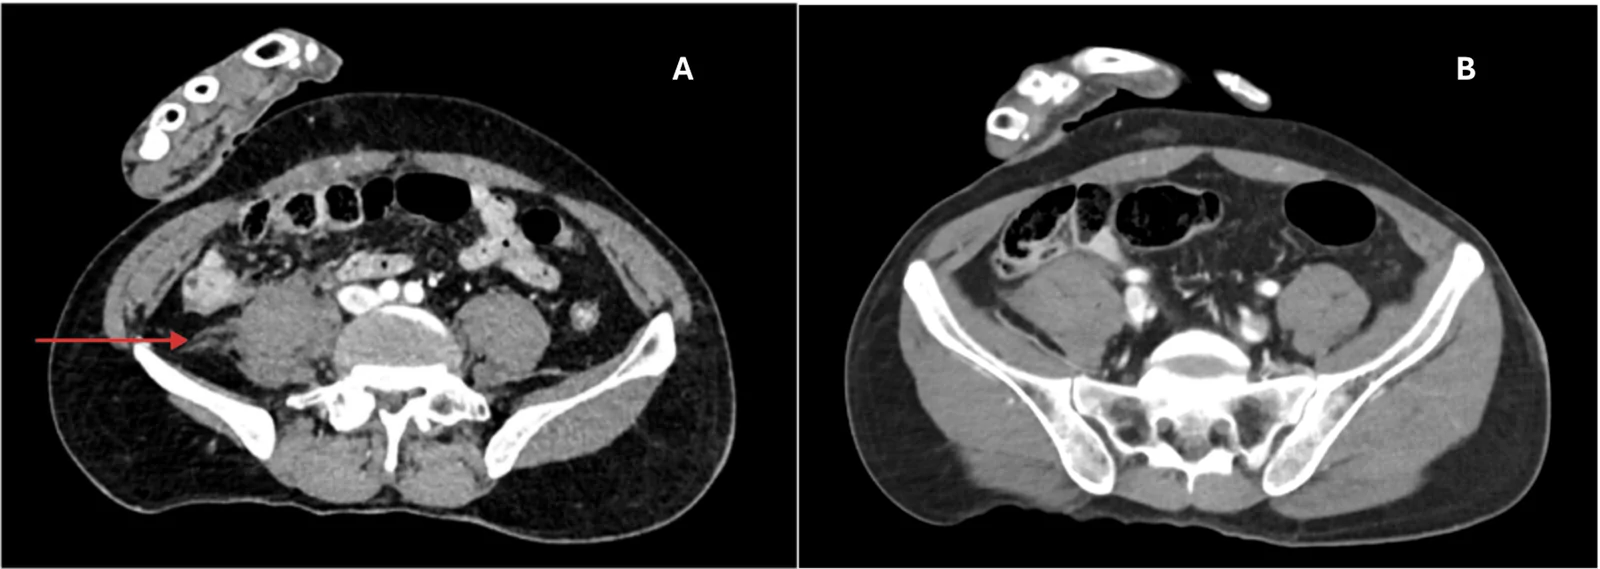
Initial CT findings on Day 0. (A) Right psoas intramuscular hematoma containing small air locules with surrounding soft tissue stranding (red arrow). (B) Representative axial CT image through the right lower abdominal/ileocecal region, with no obvious focal bowel wall discontinuity or free intraperitoneal air in this image. The initial CT examination demonstrated no pneumoperitoneum or definitive radiological evidence of bowel or mesenteric injury. The red arrow points at the right psoas intramuscular hematoma.
